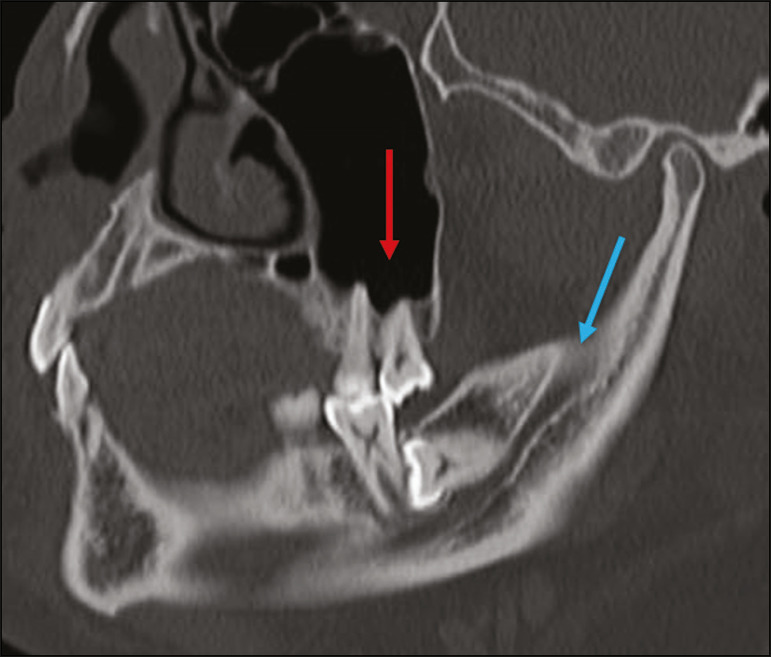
Sagittal CT of the paranasal sinuses showing the relationship between the maxillary dental arch and the floor of the maxillary sinus (red arrow), as well as the relationship between an impacted third molar (in the mandibular dental arch) and the mandibular canal where the inferior alveolar nerve passes (blue arrow).
